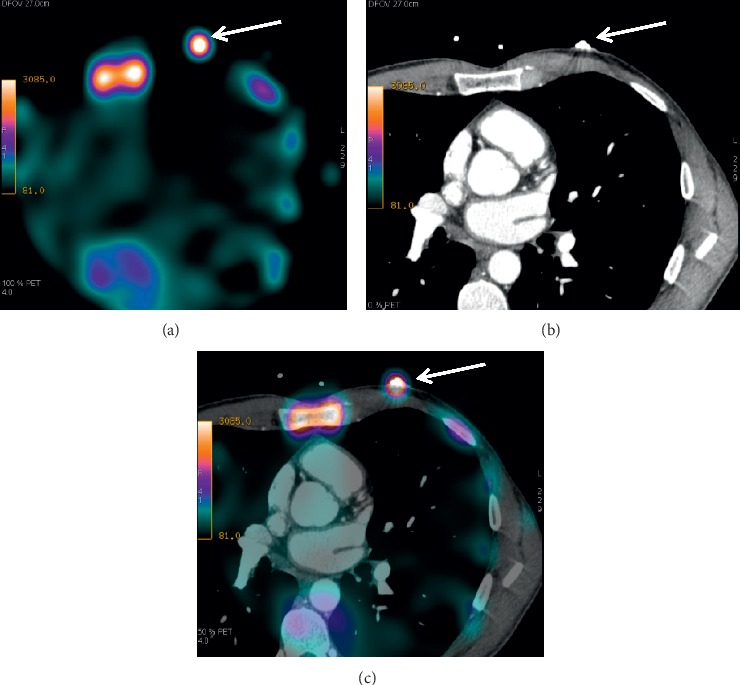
An example of a fusion of the scintigraphic and CT images based on the use of surface markings. (a) Scintigraphic image (axial slice) where arrow shows a position of the radioactive marking; (b) CT tomographic image (axial slice) where arrow shows a position of the radiopaque marking; (c) SPECT/CT image where arrow shows a superposition of two markings.
